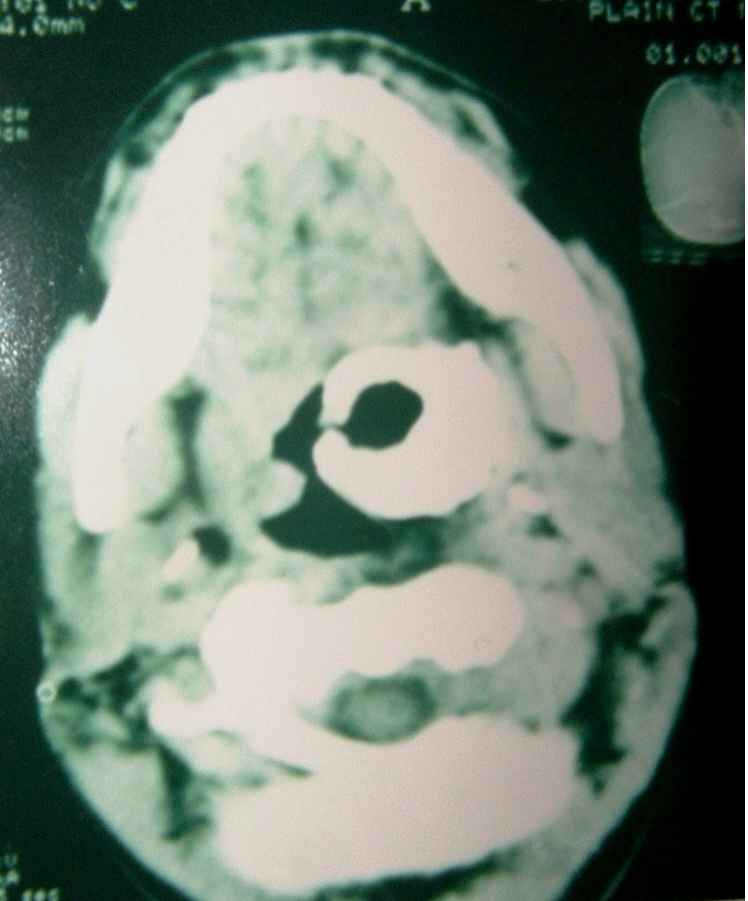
CT scan showing stone in oral cavity.
